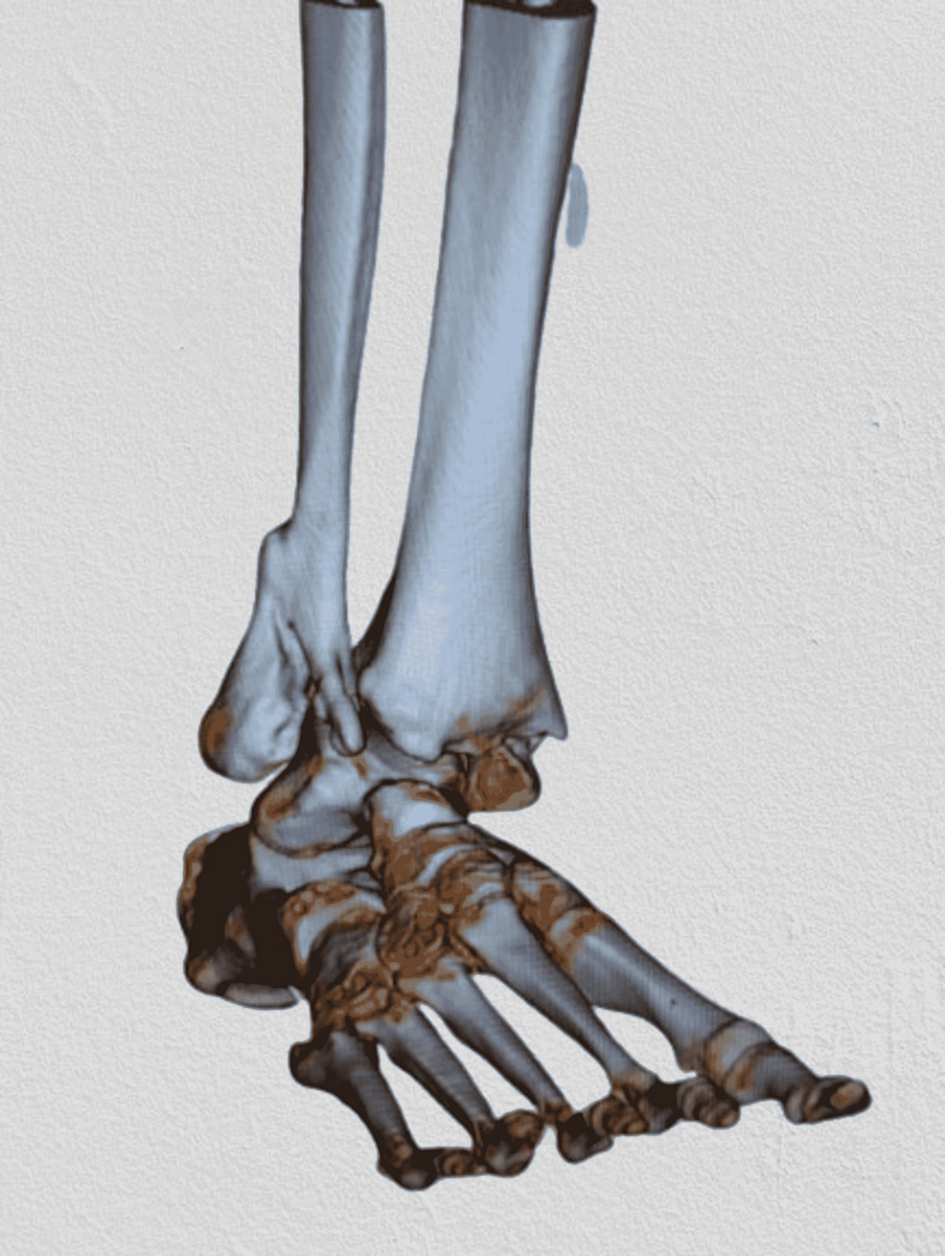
CT scan of the right ankle
